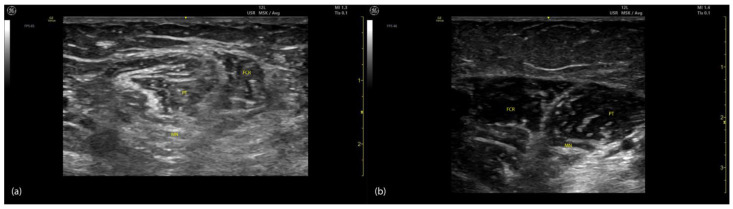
Comparison of ultrasound characteristics in spastic and normal PT and FCR: (a) ultrasound image of spastic muscles, including the PT and FCR, showing altered echogenicity and disrupted muscular architecture; (b) ultrasound image of the same muscles in a normal state, demonstrating uniform echotexture, clear muscle boundaries, and normal positioning of the MN; PT—pronator teres; FCR—flexor carpi radialis; MN—median nerve.
